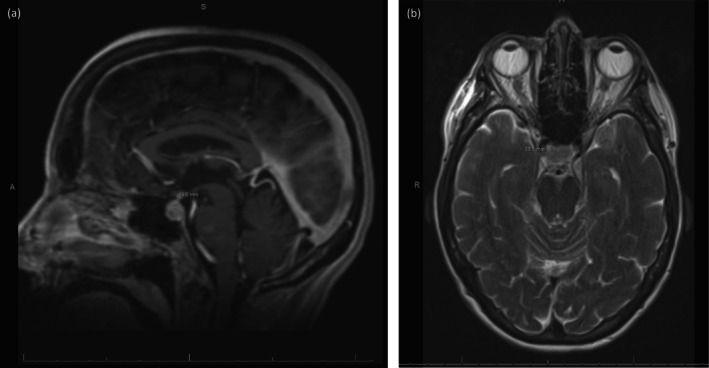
MRI Showing Pituitary Enlargement with Differential Considerations. (a) A sagittal T1‐weighted MRI image of the brain demonstrates that the pituitary gland is enlarged for age, with a convex upper border and measuring approximately 13 mm. (b) An axial T2‐weighted MRI image of the brain demonstrating hypodensity and enlargement of the pituitary gland approximately 13.1 mm in diameter. The differential diagnosis of this image alone includes adenoma, hypophysitis (associated with some immune checkpoint inhibitor), dysplasia, and metastatic disease.
